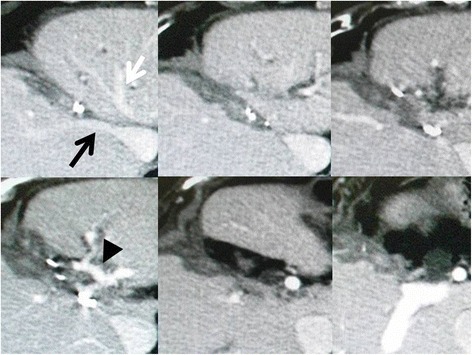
Computed tomography findings after the segment 4 segmentectomy. Although the right (*black arrow*) and left (*white arrow*) hepatic veins were recognizable, the middle hepatic vein was not observed. Furthermore, liver parenchyma right and adjacent to the umbilical portion of the portal vein (*black arrowhead*) was not identified. These findings suggested that segmentectomy 4 including the middle hepatic vein was properly achieved
